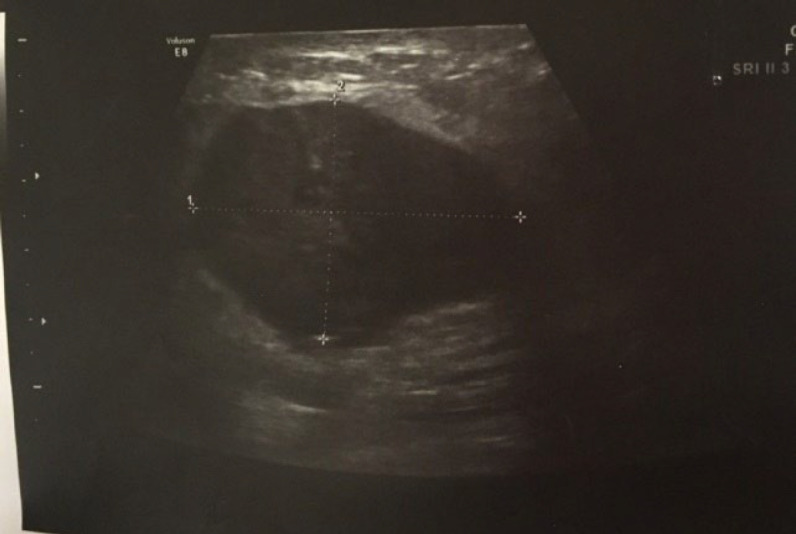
Ultrasound examination of the right breast
